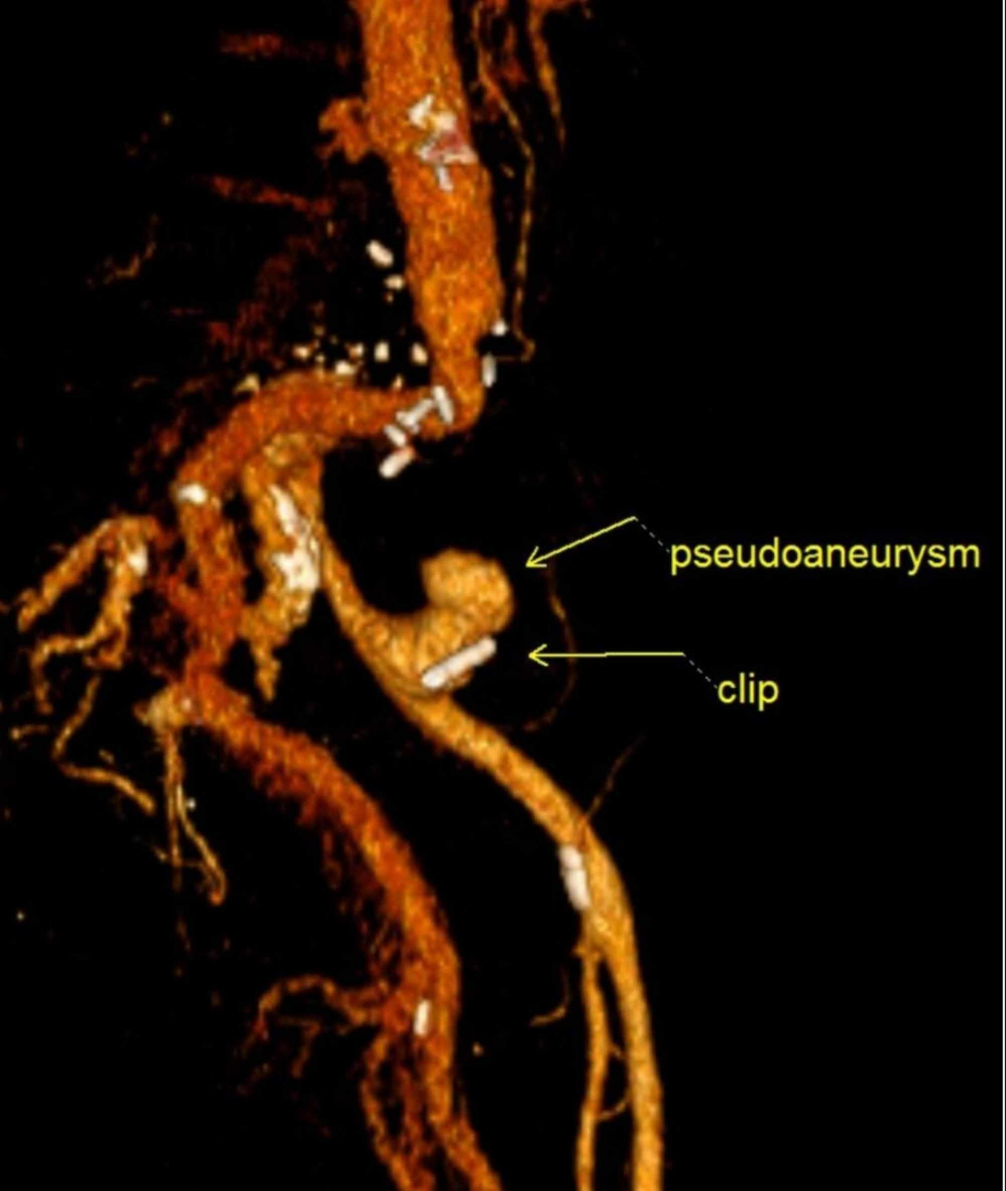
3D rendered computed tomography (CT) image demonstrates the endoscopically placed clip adjacent to the pseudoaneurysm; prior clips from previous aortoiliac surgery can also be seen
